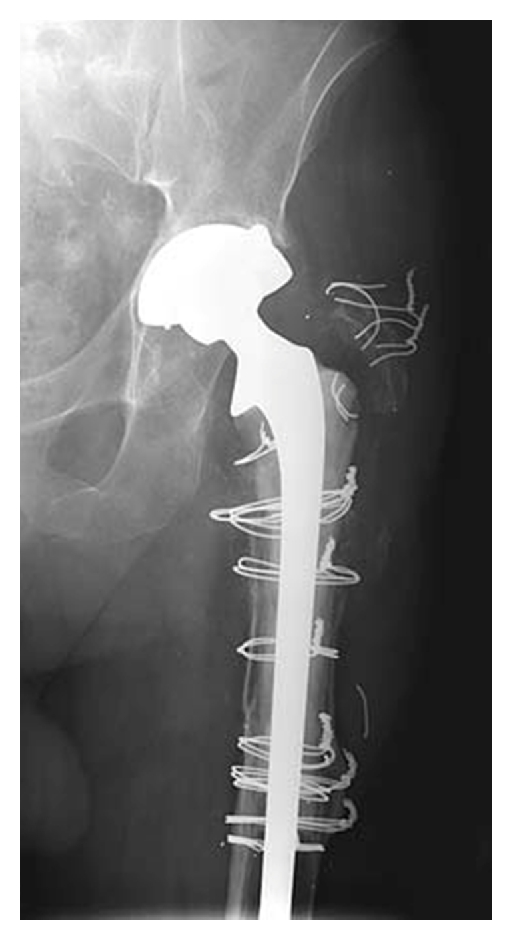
A radiograph 17 years after proximal femoral allograft (reprinted from Safir et al. [19]).
